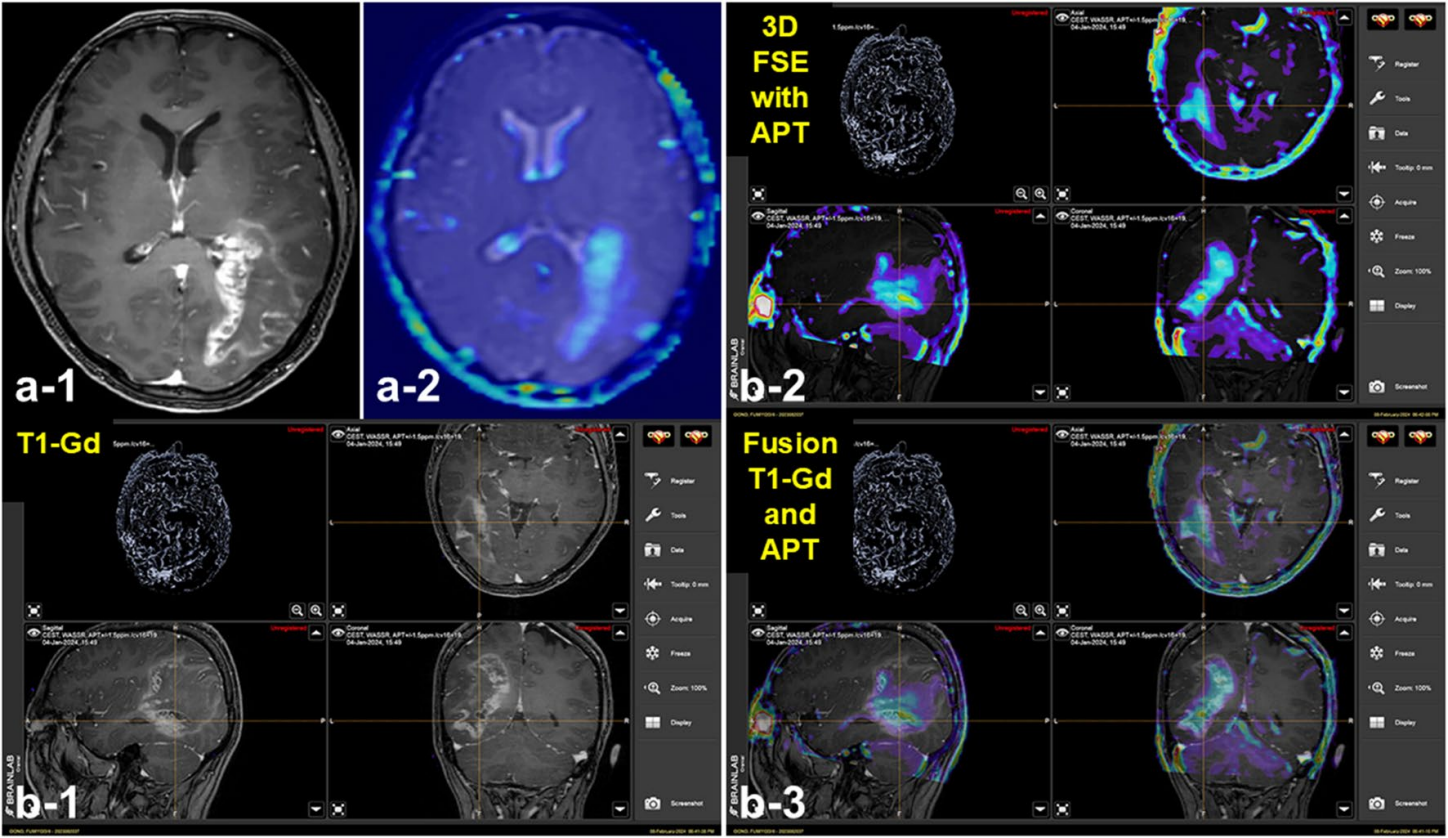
Application of 3D FSE-APT to the neuro-navigation system. Representative images from the Brainlab navigation system are shown (Patient 5). a-1) T1-Gd MRI, a-2) 3D FSE-APT, b) 3D FSE-APT-applied navigation system
